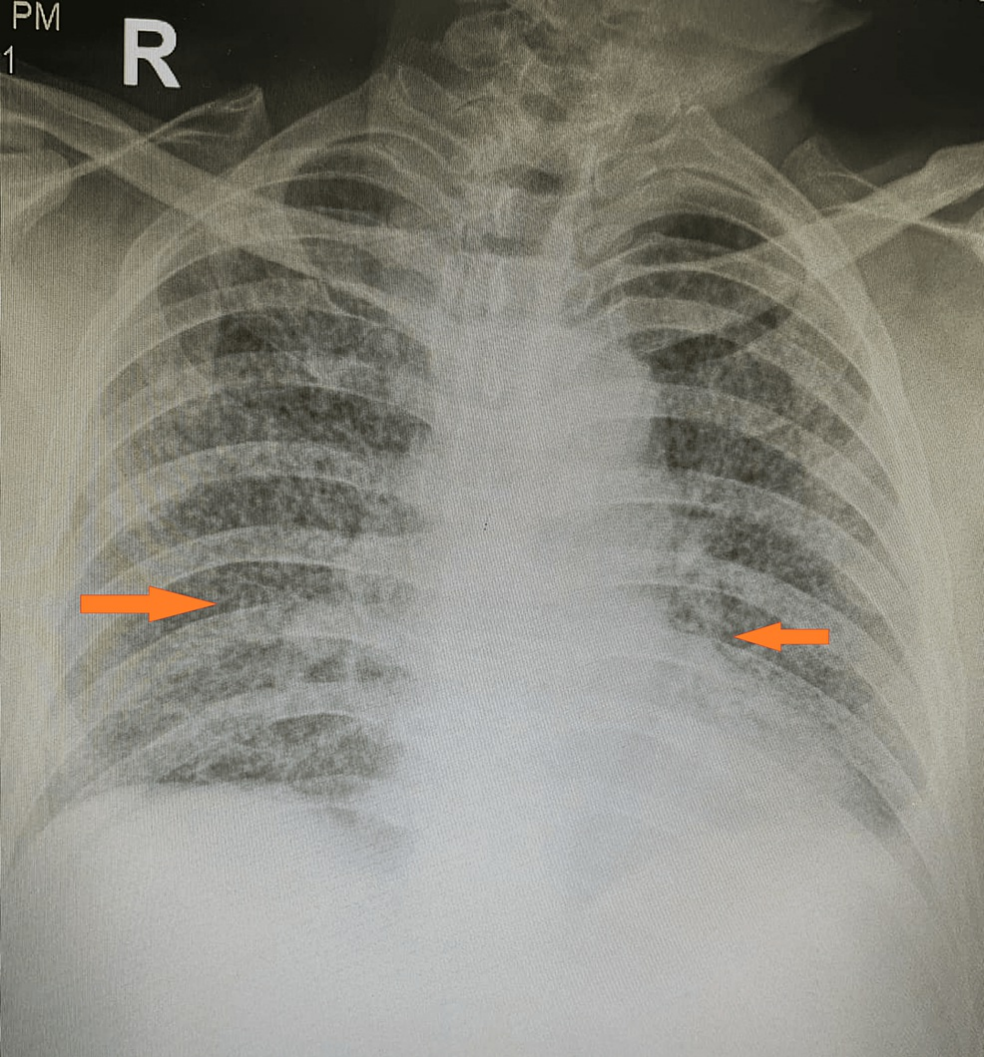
Chest X-ray showing diffuse miliary nodules throughout both lung fields
